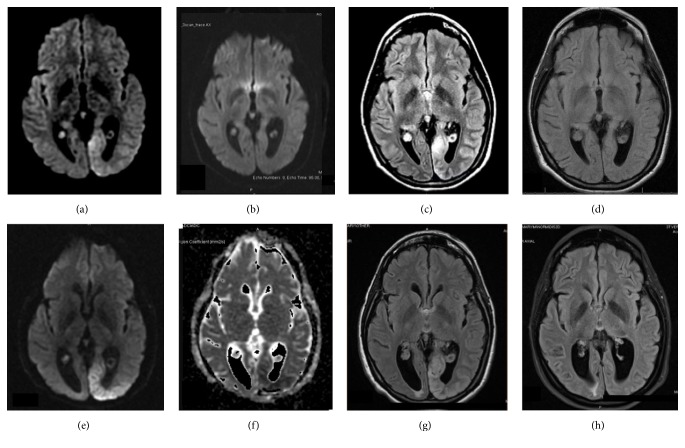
Axial brain MRI images of the patient showing transient left occipital enhancement following episode of clustered seizures in 2013 (a, b, c, d) and in 2017 (e, f, g, h). In 2013, (a) peri-ictal brain MRI-DWI shows diffusion restriction in left occipital cortex one day after her first reported seizure. (b) Follow-up MRI-DWI five weeks later shows complete resolution of abnormality in left occipital cortex. (c) Peri-ictal brain MRI T2/FLAIR shows hyperintense signal in left occipital cortex one day after her first reported seizure. (d) Follow-up MRI T2/FLAIR five weeks after seizure activity shows complete resolution of abnormality in left occipital cortex. In 2017, (e) peri-ictal brain MRI-DWI shows diffusion restriction in left occipital cortex one day after her first reported seizure. (f) Peri-ictal ADC map shows hypointensity in the left occipital cortex. (g) Peri-ictal brain MRI T2/FLAIR shows hyperintense signal in left occipital cortex. (h) Follow-up MRI T2/FLAIR six weeks following seizure activity shows complete resolution of abnormality in left occipital cortex.
